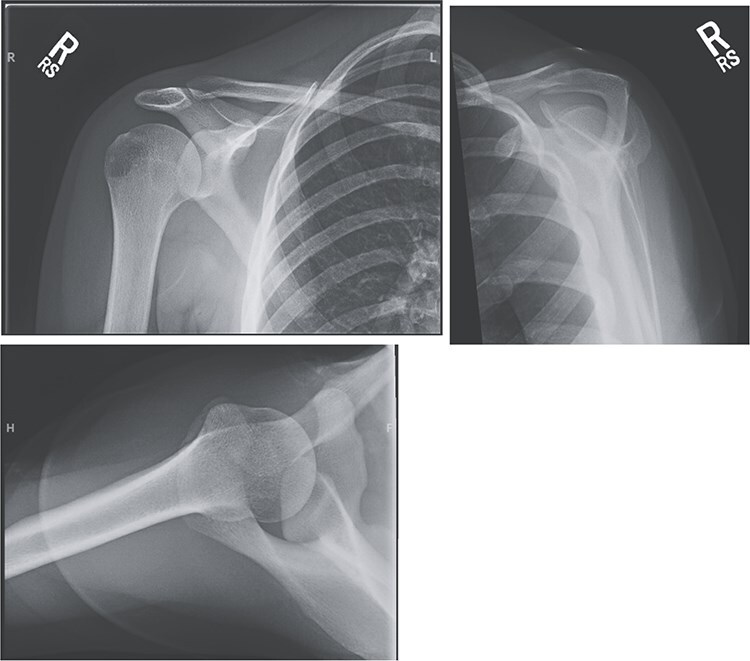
Radiographic imaging on initial presentation was without transverse scapular ligament calcification.
